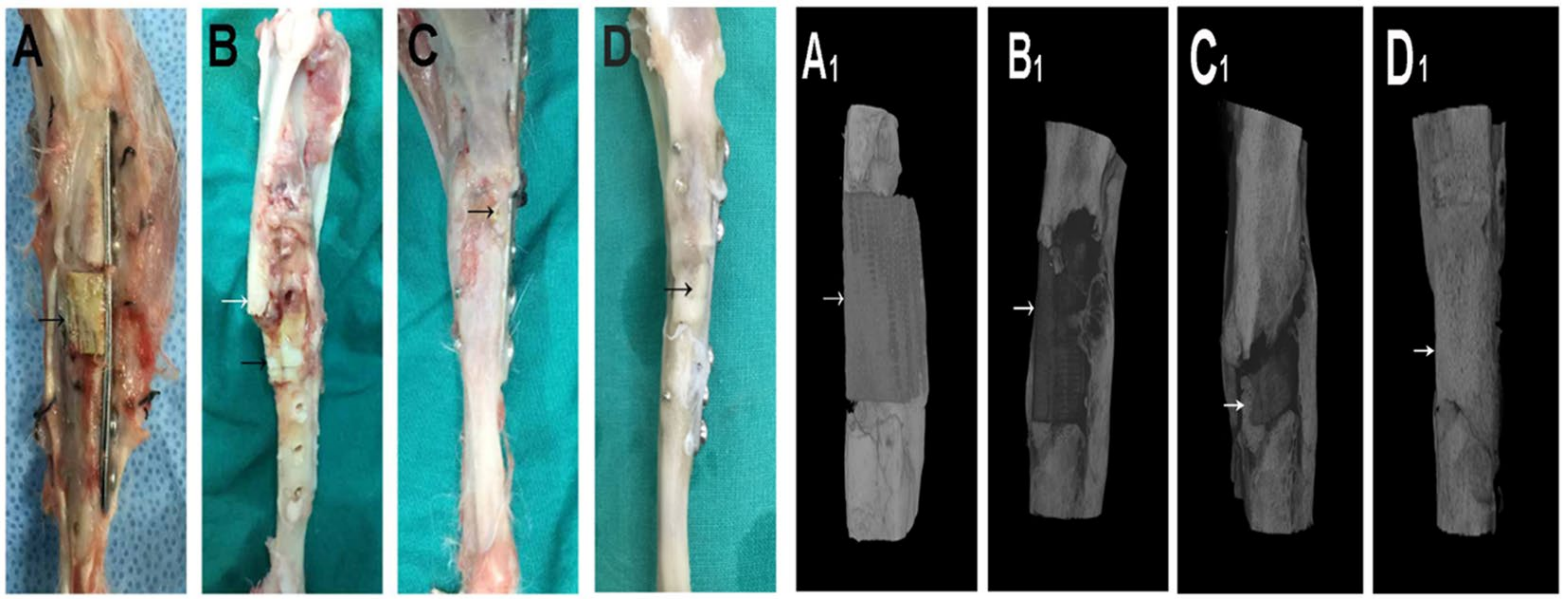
Gross appearance and CT reconstruction images of control (A,A
